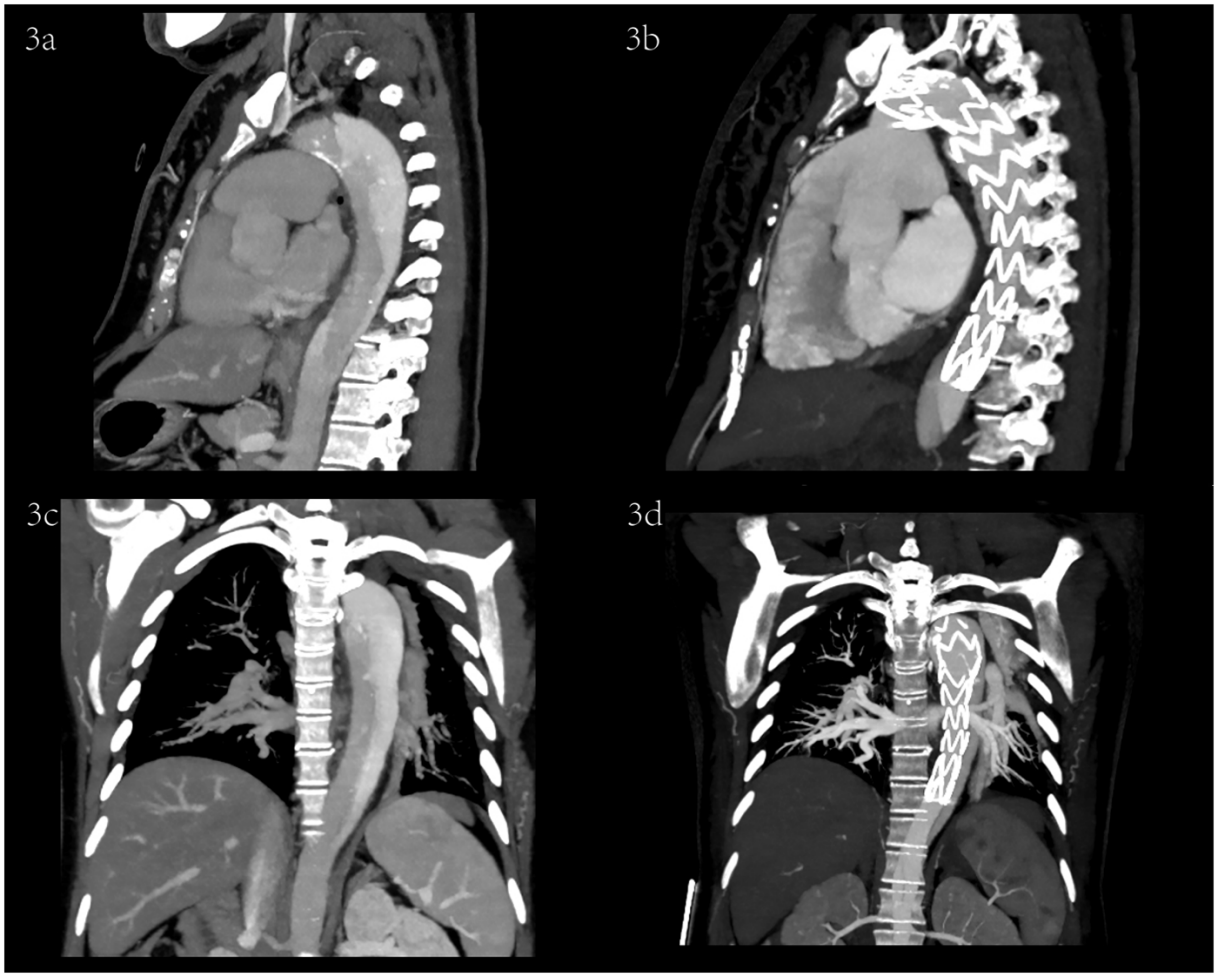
Sagittal and coronal CTA images before and after TEVAR.
